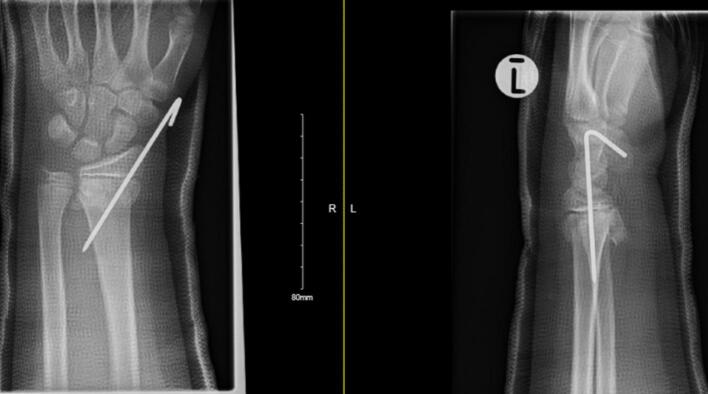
AP and lateral radiographs 5 days post-operatively.
